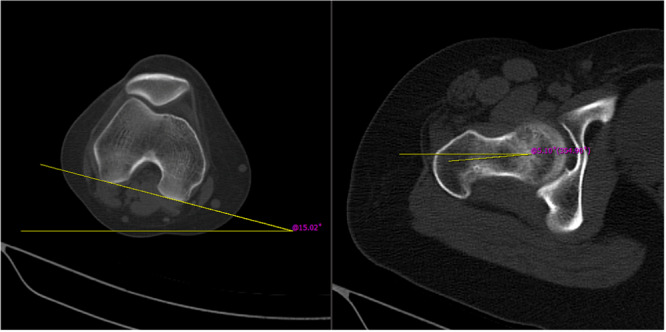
Measurement of femoral anteversion by CT scan. A line drawn from the femoral head's centre to the base of the neck's centre and another line joining the exact posterior sides of the lateral and medial condyles were used to define the femoral anteversion on CT scans (Hernandez et al. method). CT, computed tomography.
